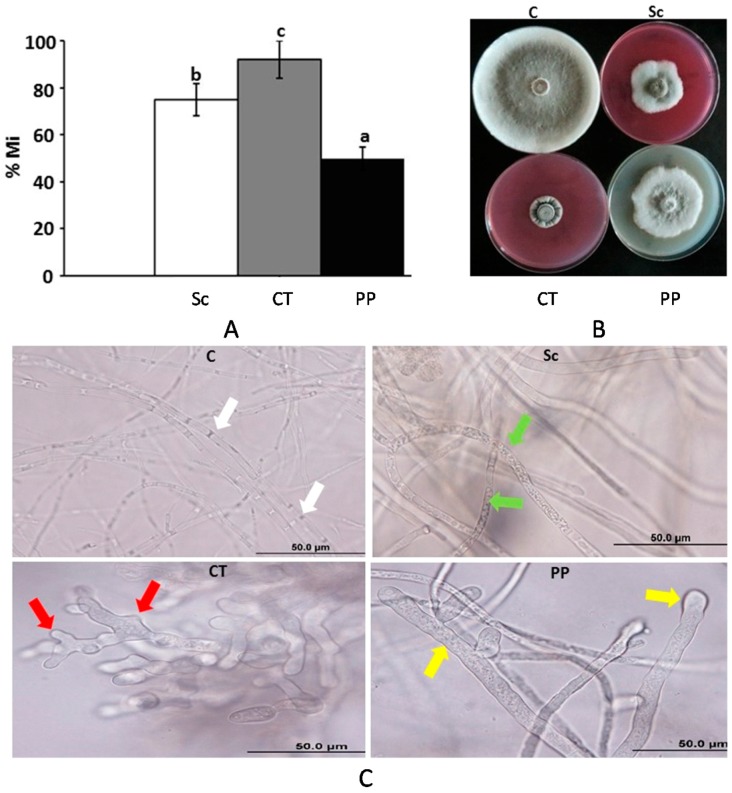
Efficacy of fungicides against *L. theobromae*. (A) Growth inhibition rate (% Mi) of *L. theobromae* using 250 ppm of the fungicides after 10 days. Values with different letters are significantly different from each other at *p* < 0.05; (B) the effect of fungicides (250 ppm) on in vitro mycelial growth; and (C) abnormalities in hyphal morphology, septum formation, and cytoplasmic contents of *L. theobromae*, following fungicide treatments, compared to control. White arrows indicate normal septate hyphal growth; green arrows indicate formation of non-septate hyphal formation and cytoplasmic coagulation; red arrows indicate hyphal swellings and branch deformation; yellow arrows indicate hyphal swellings and cytoplasmic coagulation. C, control (no fungicide); Sc, Score^®^; CT, Cidely^®^ Top; PP, Penthiopyrad^®^.
